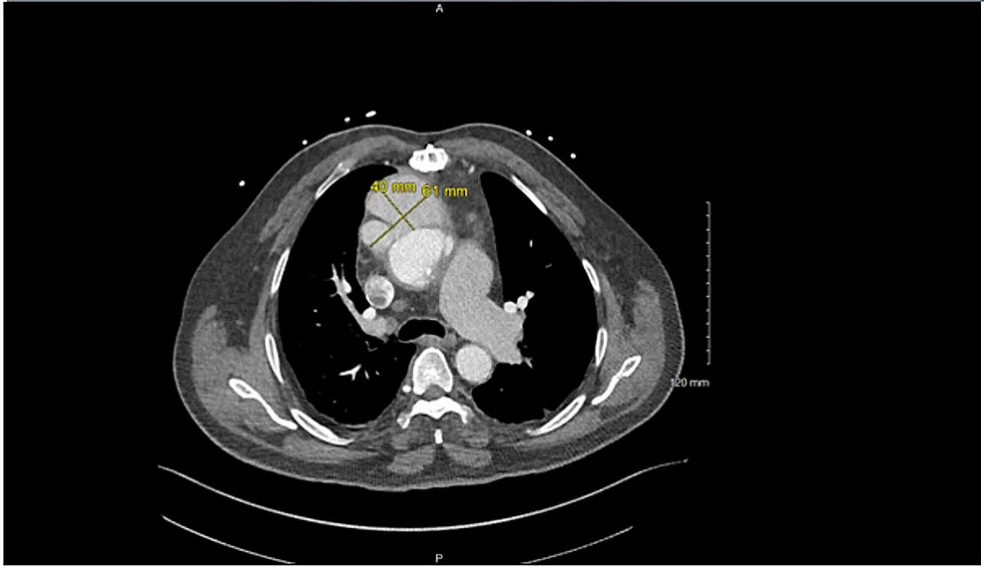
Trans-axial view of CT scan demonstrating the pseudoaneurysm of the aorta; yellow line demarcates the pseudoaneurysm with exact dimensions.
